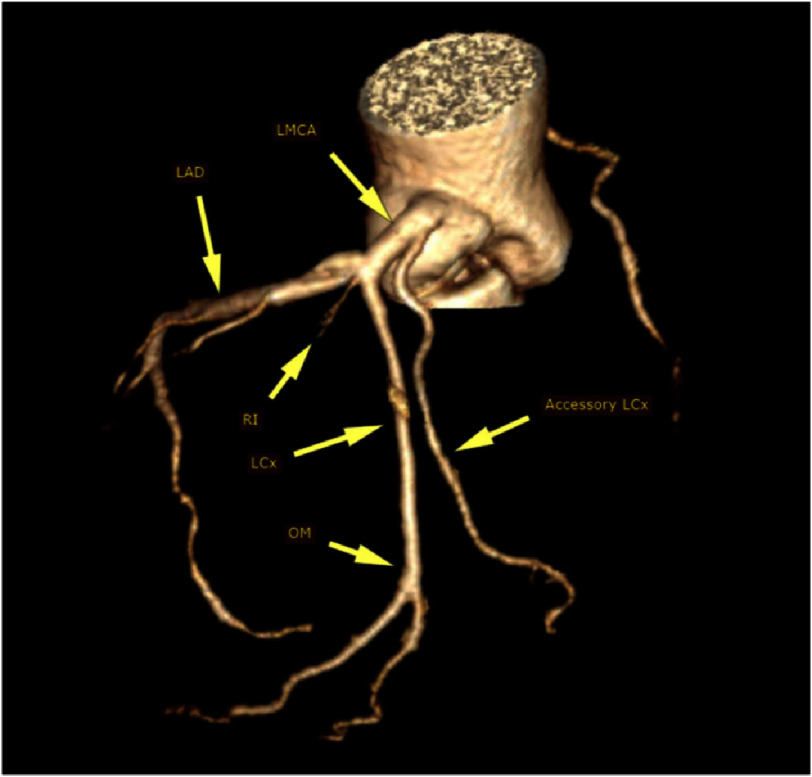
Volume-rendered computed tomography of the coronary tree showing trifurcation of left main coronary artery and acessory circumflex artery. LMCA: left main coronary artery, LAD: left anterior descending, LCx: left circumflex, RI: ramus intermedius, OM: obtuse marginal.
